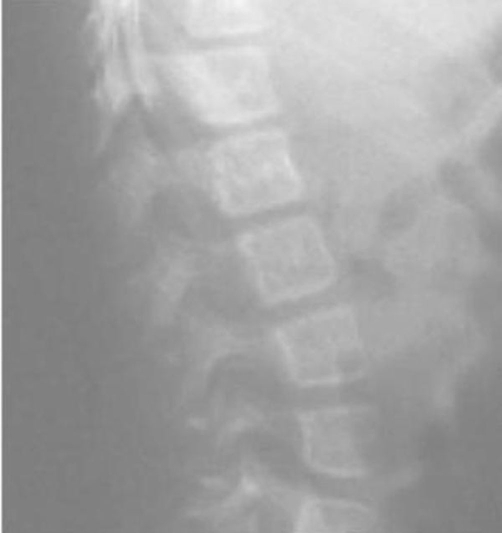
A-P spine lateral radiograph showed square shaped vertebrae.
